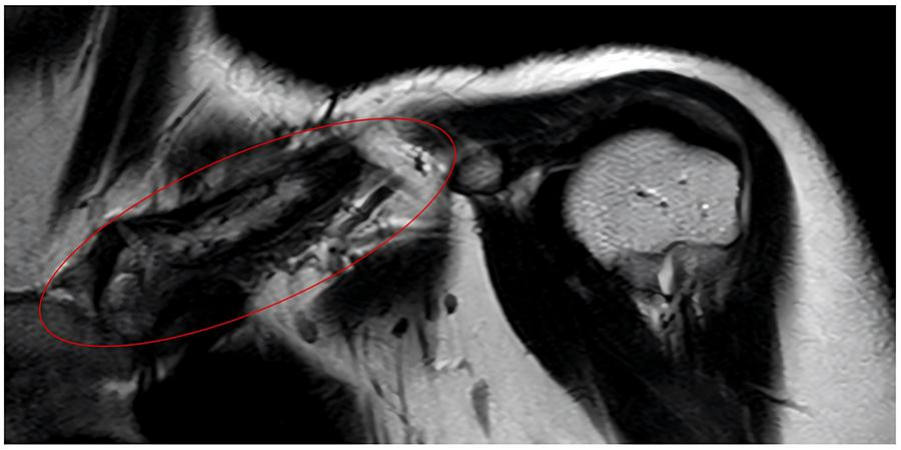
Follow-up MRI: persistent structural changes, including osteolytic destruction and periosteal reaction, are observed in the left clavicle.
